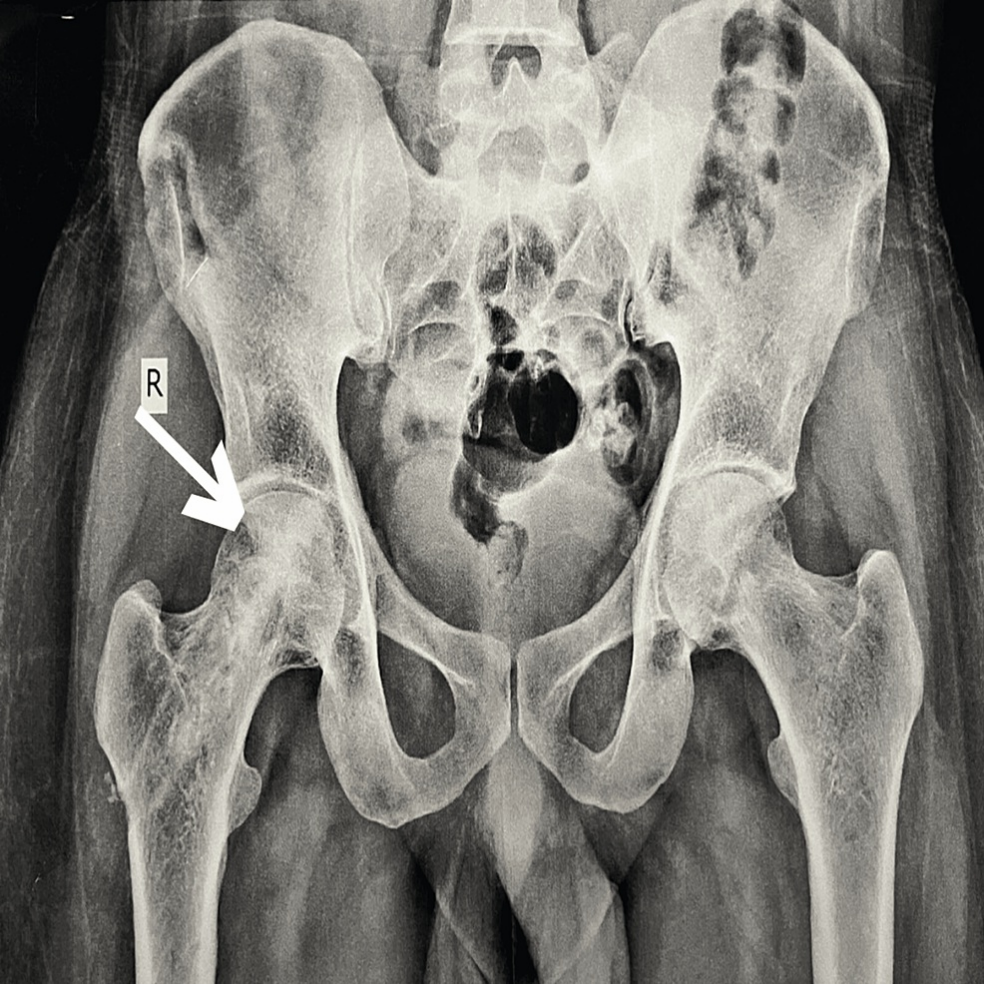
Loss of contour of right femoral head X-ray of the pelvis (anteroposterior view)
